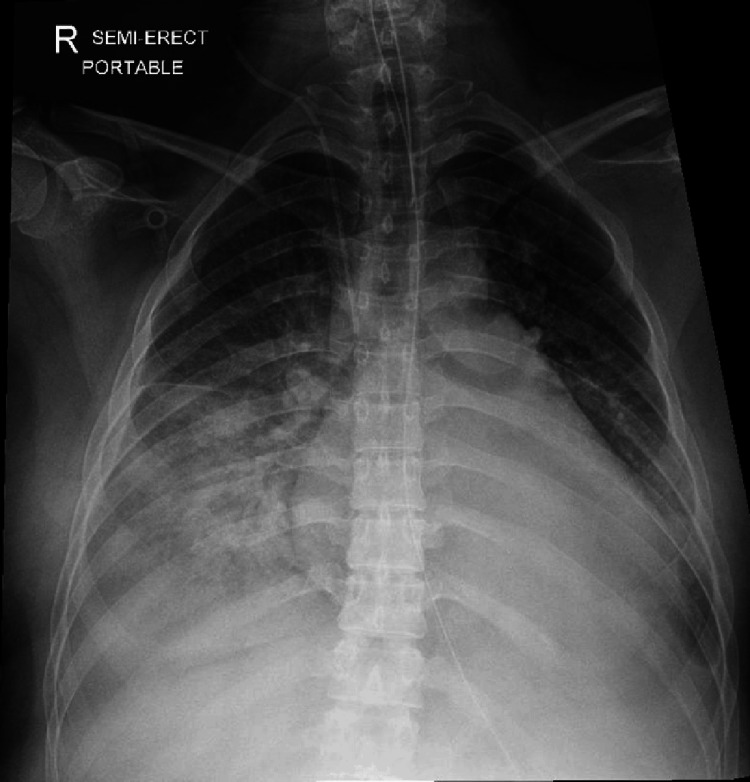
Chest radiograph taken shortly after admission to ICU Although cardiomegaly is conventionally diagnosed on a posterior-anterior chest radiograph, this anterior-posterior film suggests cardiomegaly even after considering projection differences, especially in this context of features indicative of pulmonary oedema (severe air space shadowing in bilateral lower zones and upper lobe diversion) ICU: intensive care unit
